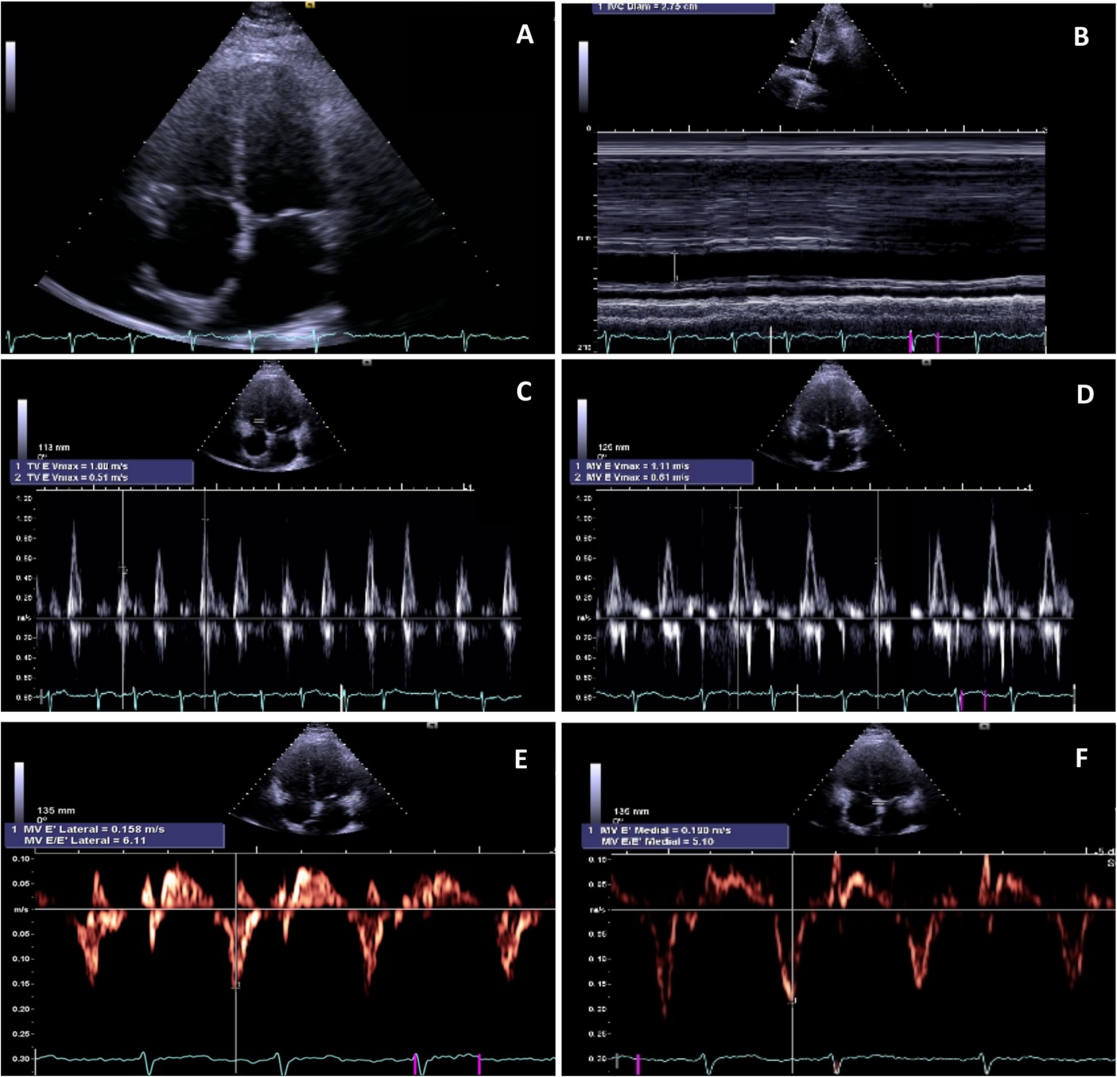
Transthoracic echocardiography performed during hospital stay. A Left and right atrial enlargement, pericardial thickness and effusion. B Inferior vena cava plethora. C, D Marked tricuspid and mitral valves inflow respiratory variations. E, F Pulsed wave tissue Doppler positioned at the lateral and septal mitral annulus
